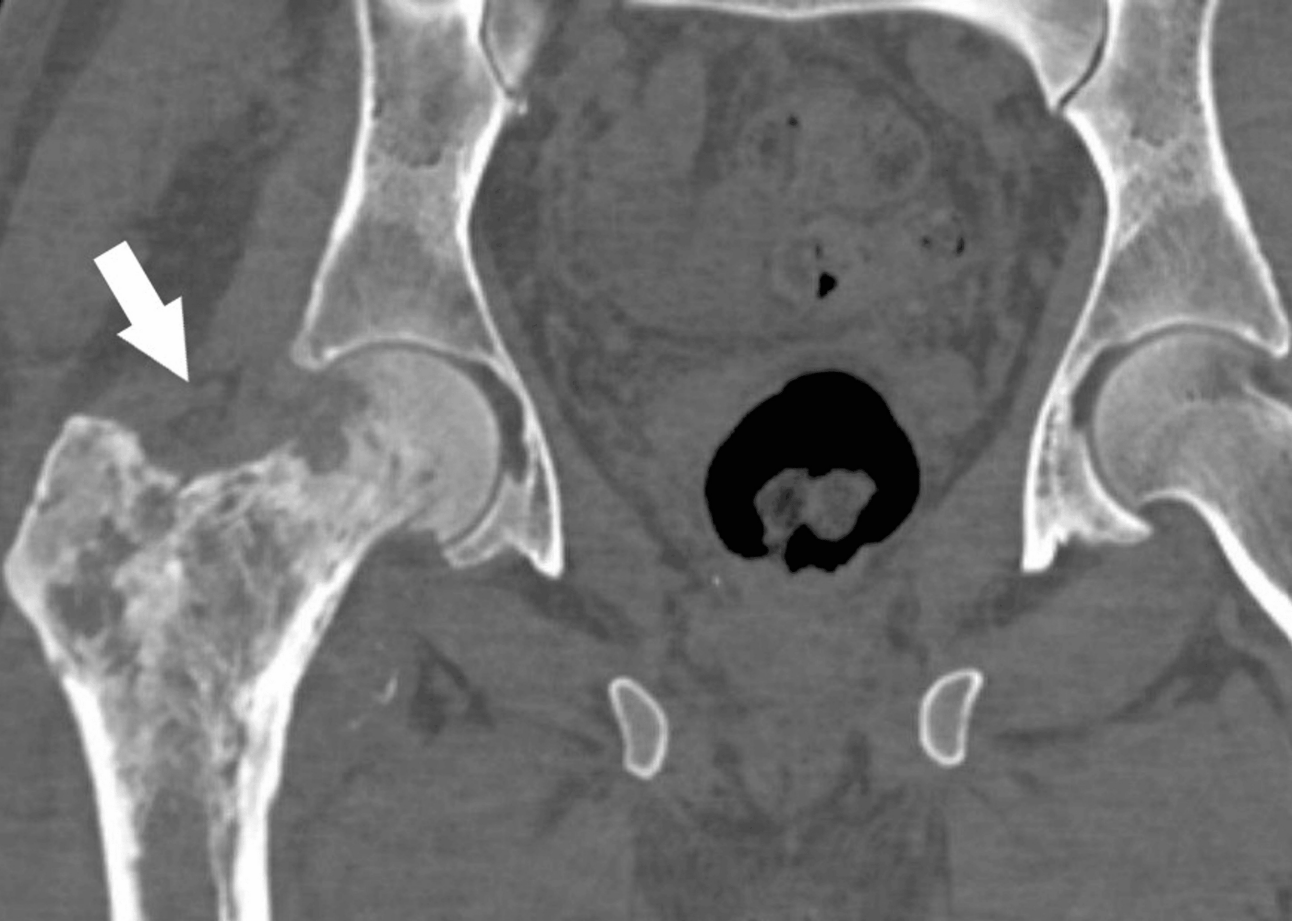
Coronal pelvic CT scan showing secondary lesions of the right femoral neck.
